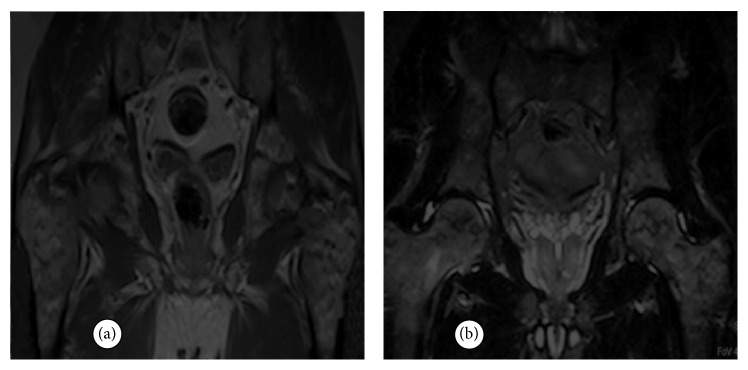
MRI scan of pelvis and both femurs showed multiple metastatic bone lesions dominantly located in both femoral heads, necks, and trochanters with a largest of diameter 2 cm. (a) Multiple hypointense metastatic bone lesions on T1-weighted coronal imaging. (b) Multiple hyperintense metastatic bone lesions on T2-weighted coronal imaging.
